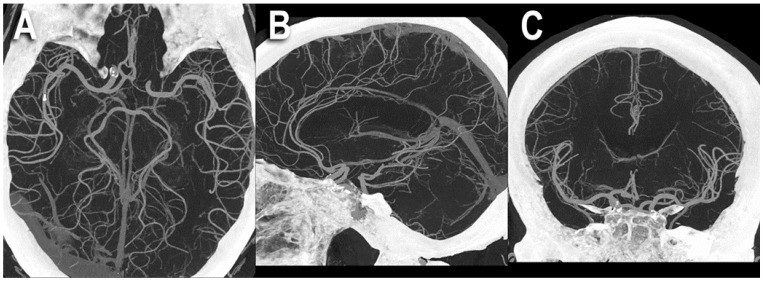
PCCT angiography of the head: MIP volumetric display. The figure shows a PCCT angiography of the brain with ultra-high resolution of 100 microns (source dataset; matrix 1024 × 1024; slice thickness/increment 0.2/0.1 mm; convolution kernel B60f; radiation dose comparable to equivalent CT angiography of the head with comparable Dual-source CT of the 3rd generation) using thick-slab 3D Maximum Intensity Projection (MIP) in the three main planes: axial (A), sagittal (B), and coronal (C). To be noted is the massive increase in the density of the small arterial vessels displayed and the length of the segments visualized; for arteria cerebralis posterior and media in (A), for arteria cerebralis anterior in (B), and for arteria cerebralis media in (C).
